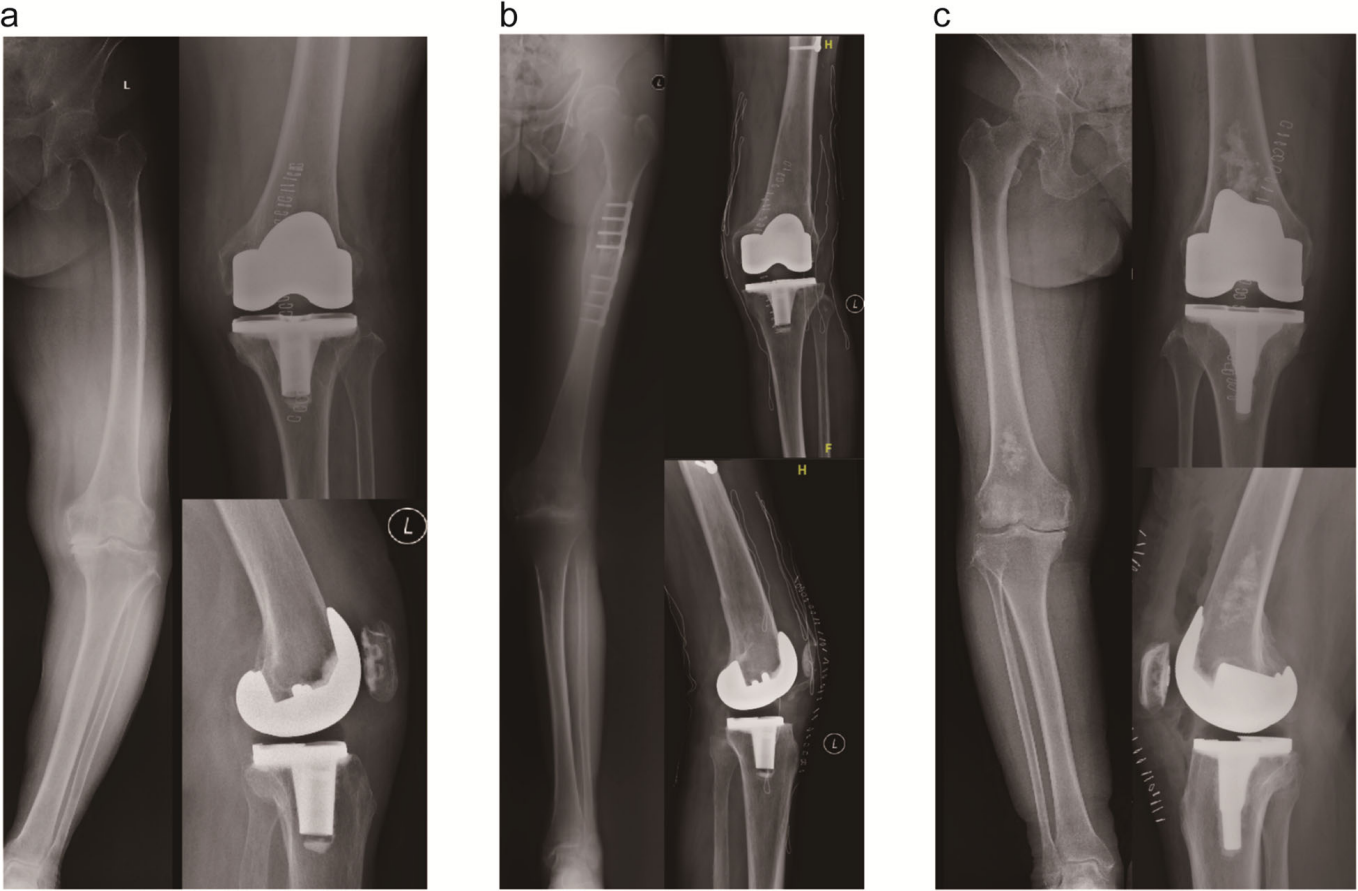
a Pre- and postoperative radiographs of patient with femoral EAD. b Pre- and postoperative radiographs of patient with lower limb fracture malunion and hardware retaining. c Pre- and postoperative radiographs of patient with benign bone tumor in distal femur
